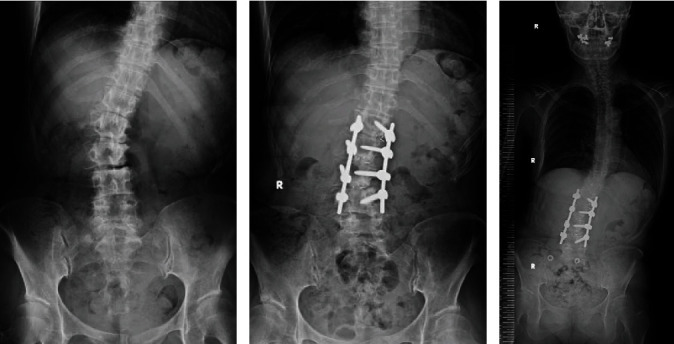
Radiographs of total spinal alignment. (a) Preoperative radiograph revealing lumbar spinal alignment in a supine position. (b) Postoperative radiograph of L1-L4 PLIF revealing lumbar spinal alignment in a supine position. (c) Radiograph showing total spinal alignment in a standing position. Pisa syndrome: the leaning of the body exacerbated when walking or standing but improved in the supine position.
